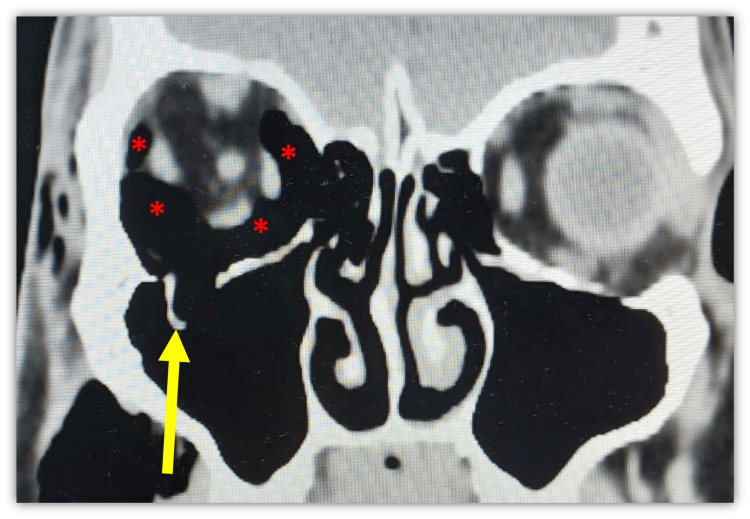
Coronal CT orbit showing right extra-conal orbital emphysema (red asterisks) with a right inferior blowout fracture (yellow arrow) CT: Computed tomography
